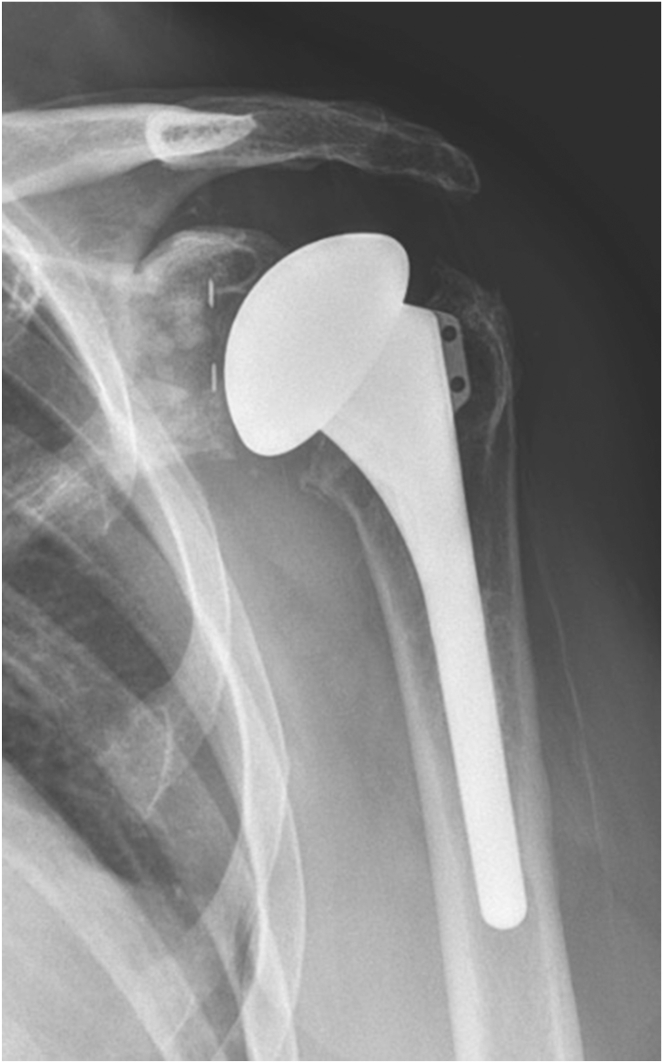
Pre-operative true anteroposterior radiograph of the shoulder with a failed anatomic total shoulder arthroplasty with a loose glenoid component.
